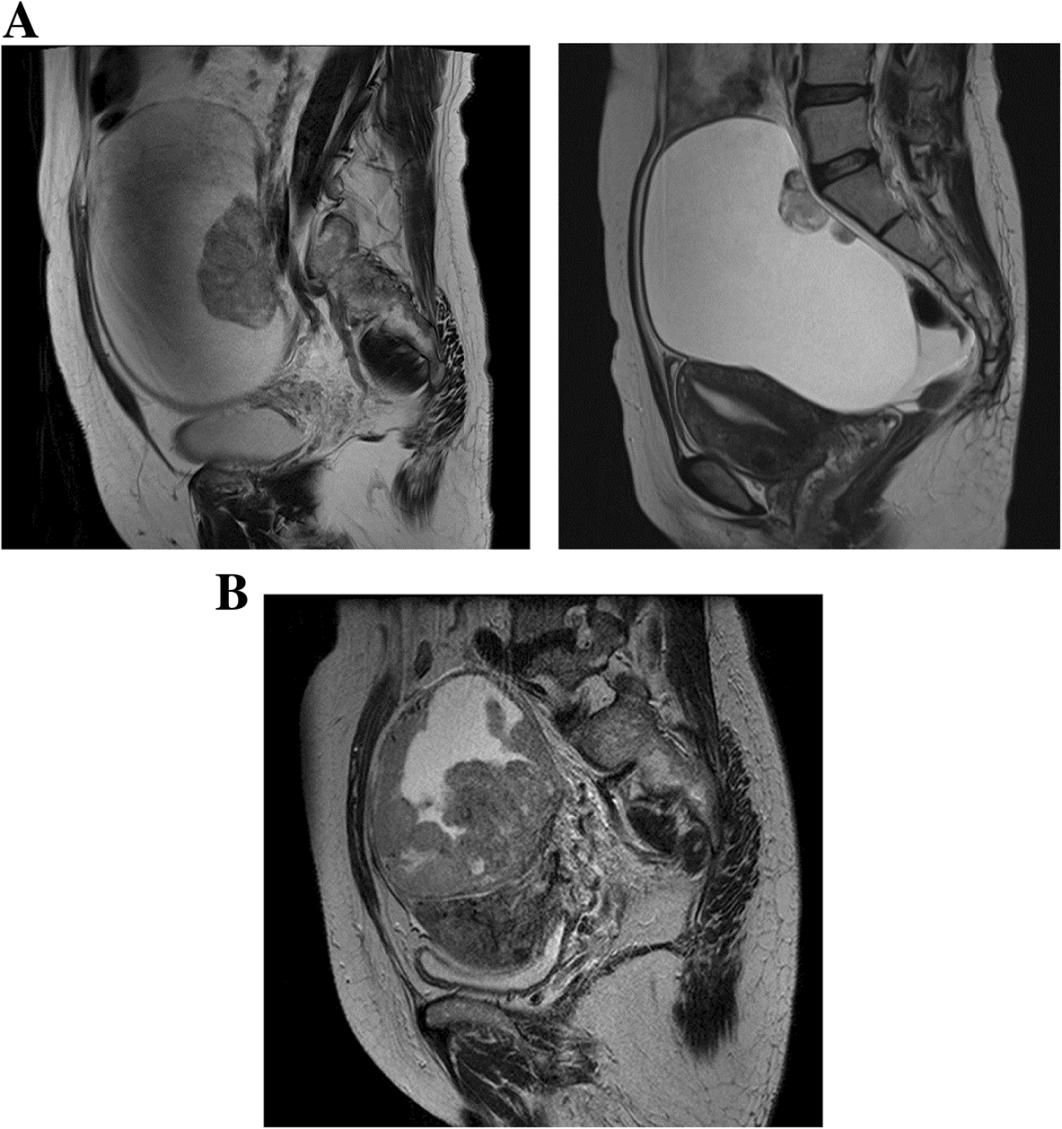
MRI features typical of CCC (a) and EC (b) lesions. T2-weighted MRI shows examples of CCC mural nodules with a focal, eccentric, or polypoid structure pattern (a) and EC mural nodules with a multifocal, concentric, or broad-based nodular pattern (b). The multifocal or concentric pattern shows the continuity of each mural nodule. MRI, magnetic resonance imaging; CCC, clear cell carcinoma; EC, endometrioid carcinoma
